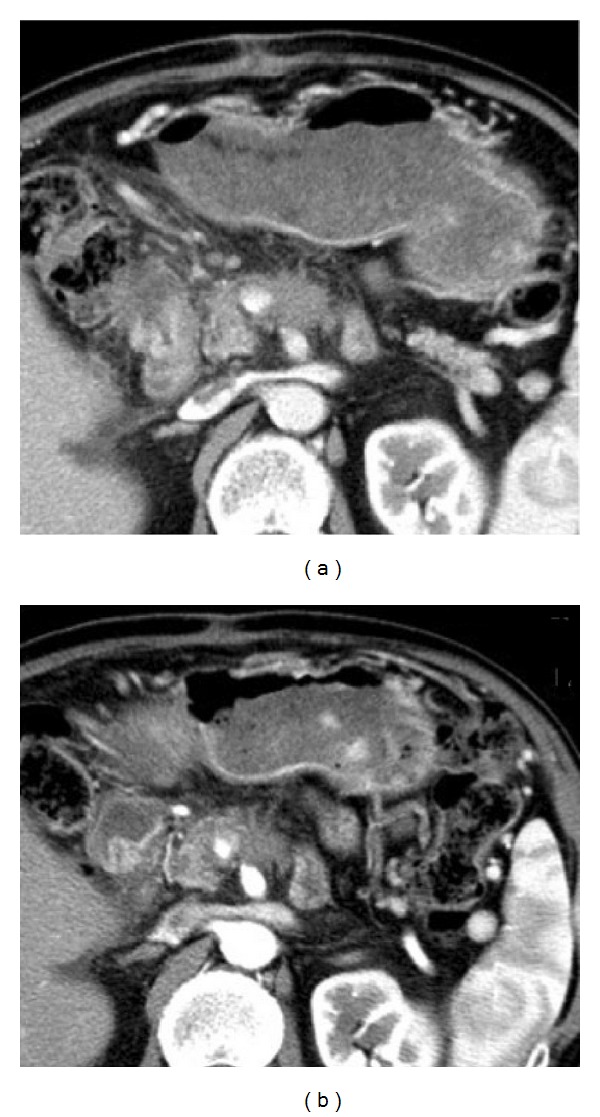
CT imaging shows no apparent change of pancreas (a) before and (b) after HIFU therapy (used with permission [18]).
